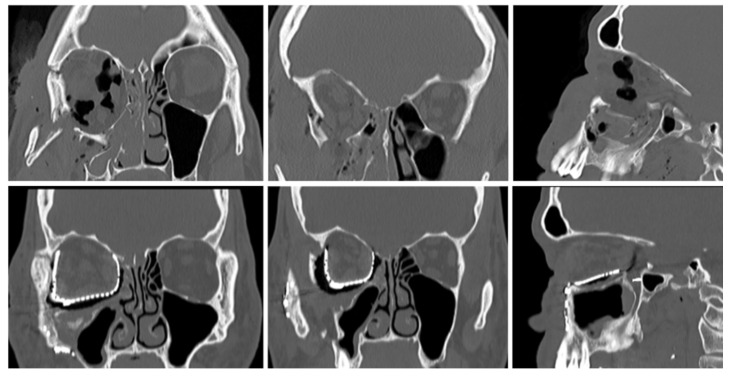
Pre and post-operative computed tomography scans showing reconstruction of the three-walled orbital fracture. In this case, the reconstruction result was assessed as “ideal”.
